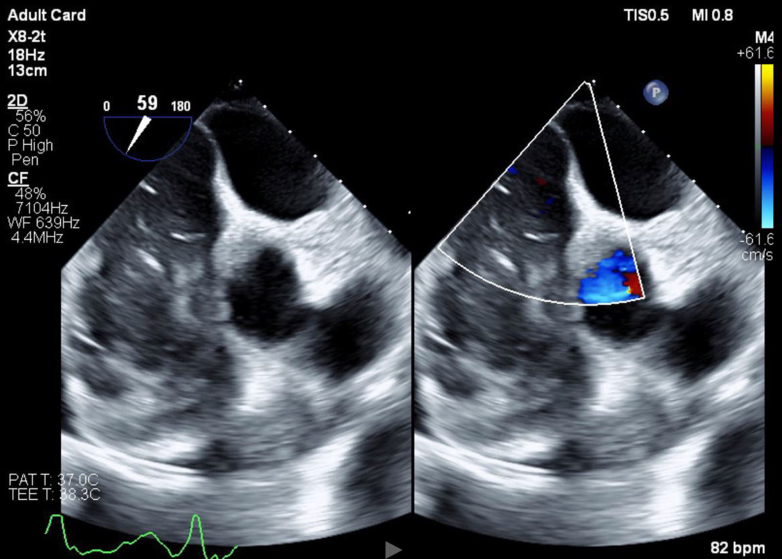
Transesophageal Echocardiogram in the Short-Axis View Demonstrating a Large, Immobile, Heterogenous, Right Atrial Mass With No Visualization of the Tricuspid Valve and Encroaching on the Superior Vena Cava The mass appears echogenic and irregular, raising suspicion for lymphomatous infiltration. The right ventricle (RV) is partially visualized.
